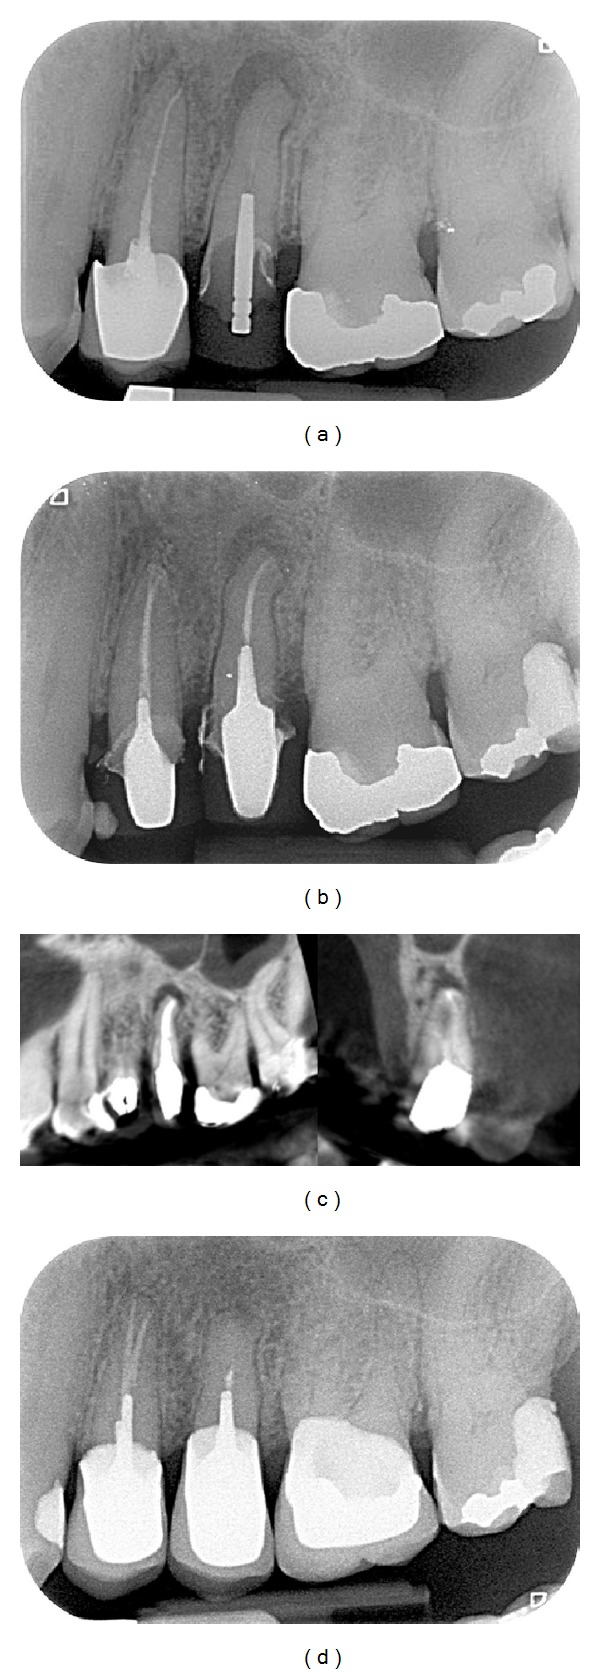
Contribution of the CBCT in the decision making process. (a) Periapical preoperative X-ray. Patient referred for the endodontic retreatment of 25. Note the periapical radiolucency on 25. (b) Control 12 months after the retreatment of 25. There are symptoms and persistent radiolucency on 25. The tooth was classified as “failure”. (c) CBCT coronal and sagittal slides of 25: a periapical surgery is indicated, (d) X-ray control 12 months after apical surgery and apical retrofilling with Biodentine (Septodont) which has the same radio-opacity as the root dentin.
